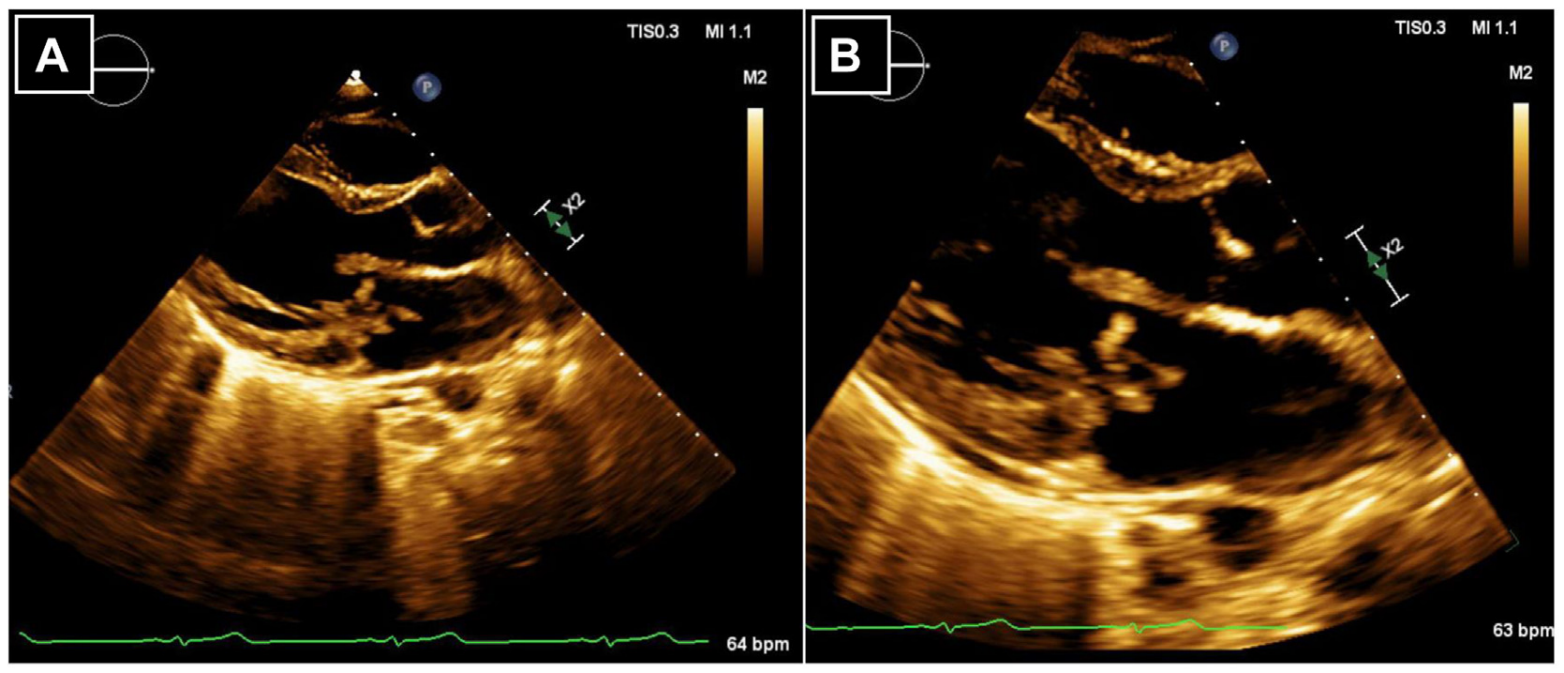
Echocardiogram. Representative images (A-B) of the echocardiogram demonstrating a large mobile multilobulated echodensity on the posterior mitral valve leaflet, measuring up to 2.5 centimeters in length.
